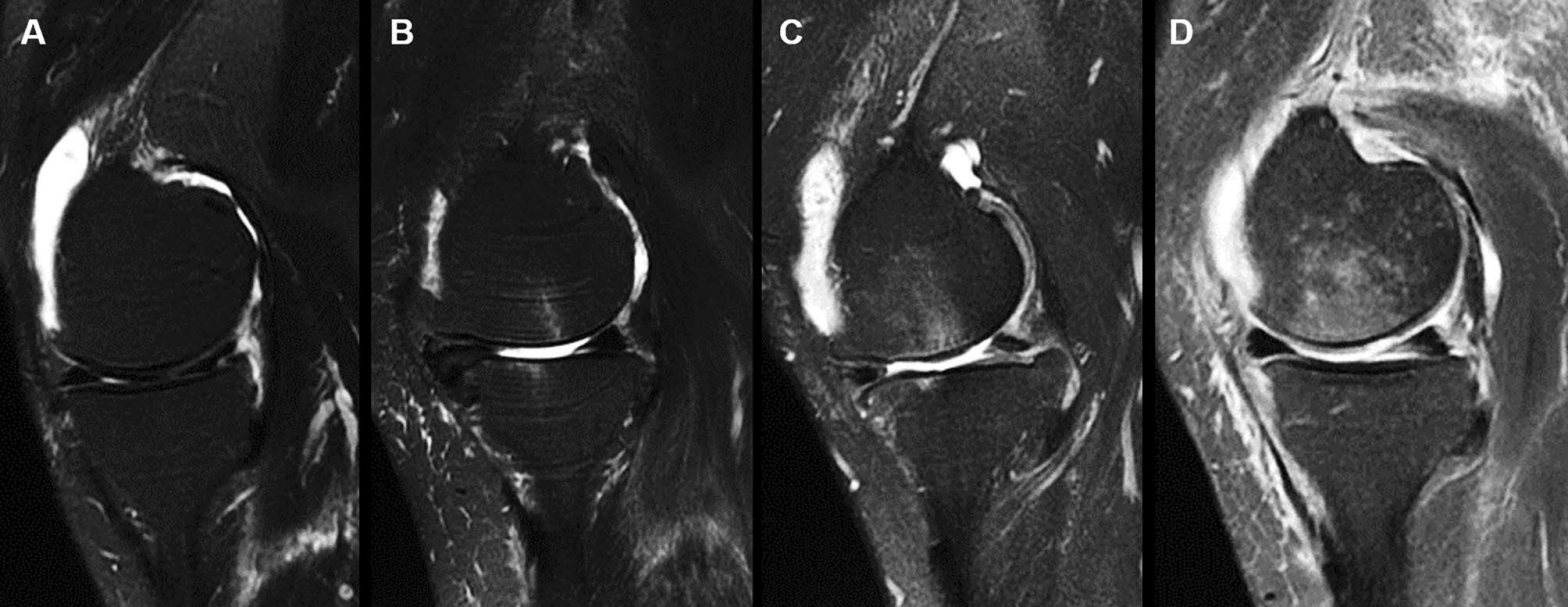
Bone marrow lesion scores using magnetic resonance imaging. Sagittal and coronal views of short TI inversion recovery (STIR) images of the knee are taken. Bone marrow lesions (BMLs) are scored using the whole magnetic resonance imaging score, where A is scored as grade 0, B as grade 1, C as grade 2, and D as grade 3 in the medial femoral condyle.
